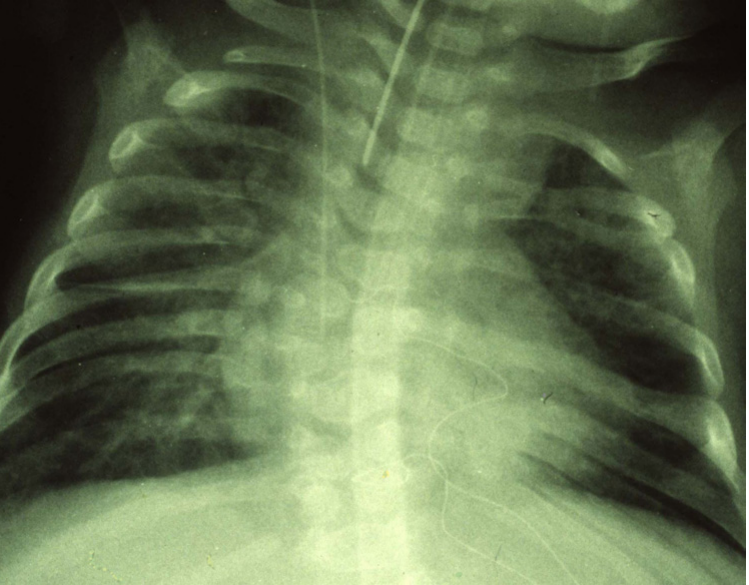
Chest x-ray of case 3 who died of Fallot's tetralogy. The picture exhibits vertebral and rib malformations and fusions along with thoracic scoliosis.
